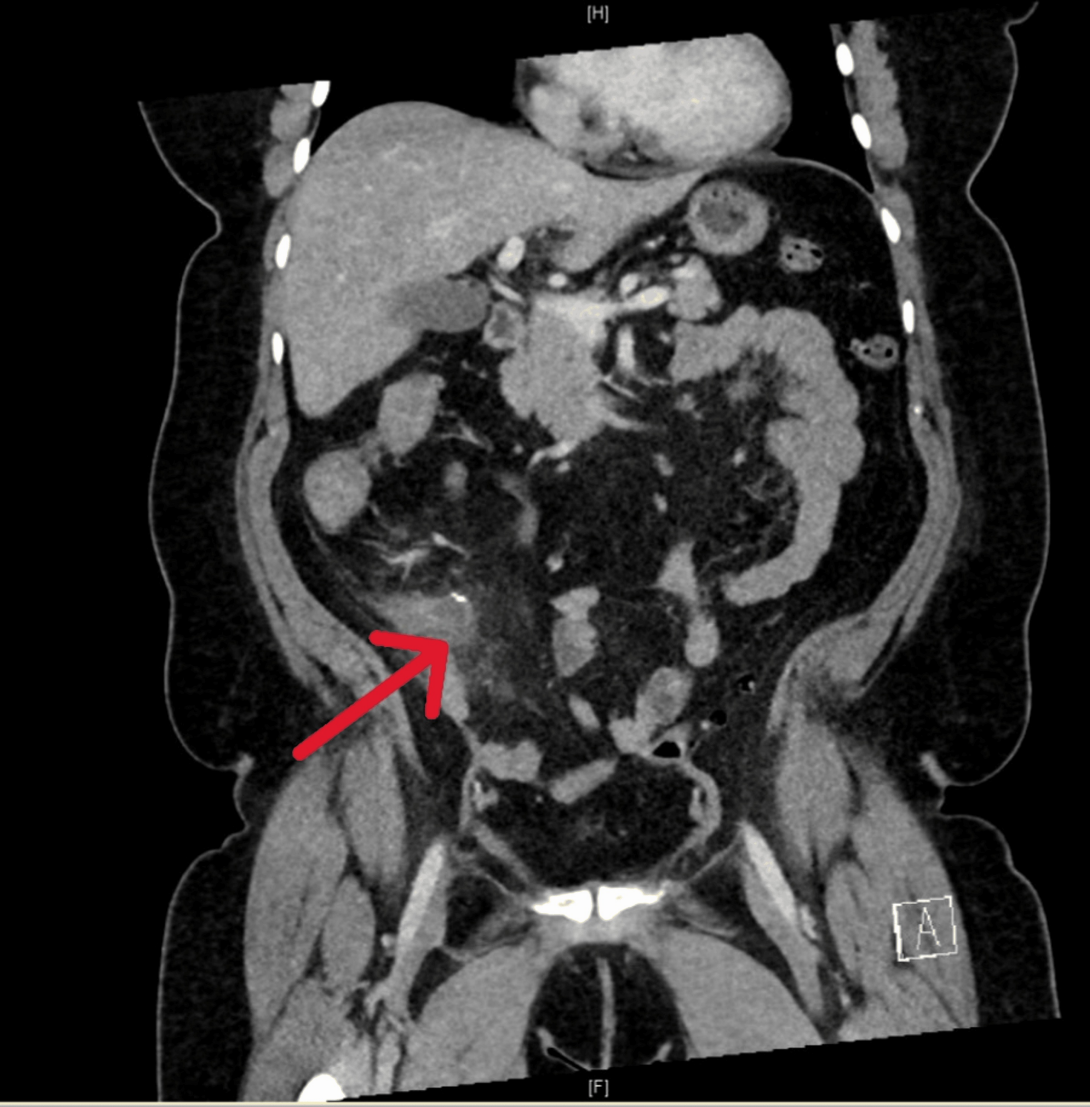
Computed tomography of abdomen and pelvis showing pericaecal image
